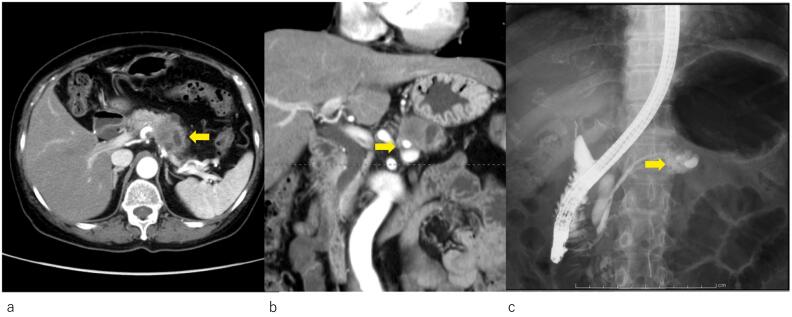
Images of the primary lesion a) Abdominal contrast-enhanced CT. A tumor shadow was noted in the distal pancreas (arrow). b) Abdominal contrast-enhanced CT. Infiltration to the splenic artery and vein was suspected (arrow). c) Endoscopic retrograde cholangiopancreatography. The main pancreatic duct was dilated to a maximum diameter of 3.2 mm, and its image terminated in the pancreatic body (arrow).
